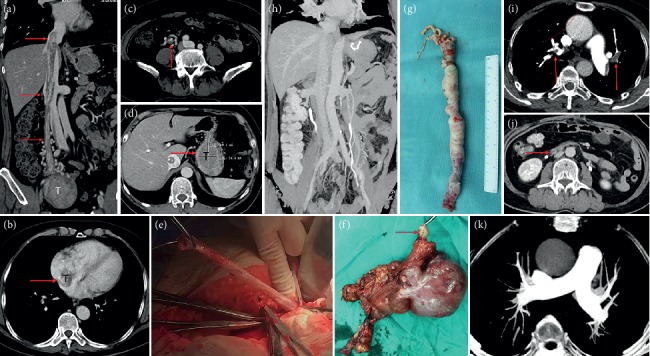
Case 4. (a) CT shows that the tumor enters into the inferior vena cava/right atrium through the right ovarian vein. (b) Right atrial tumor. (c) Arrow shows ovarian intravenous filling defect. (d) Intragastric tumor (leiomyoma was considered preoperatively); (e) the tumor is pulled out from the right ovarian vein. (f) Uterus and bilateral attachments, multiple tumors protrude from the uterine wall and the largest is 8 cm in diameter; the arrow refers to the ovarian intravenous tumor; (g) ovarian vein-inferior vena cava-right atrial tumor; (h) postoperative CT examination reveals unobstructed inferior vena cava. (i) CT shows bilateral pulmonary embolism at 10 days after surgery. (j) Right ovarian vein stump is filled with thrombus (arrow). (k) CT at 3 months after surgery shows that pulmonary artery thrombosis disappeared.
